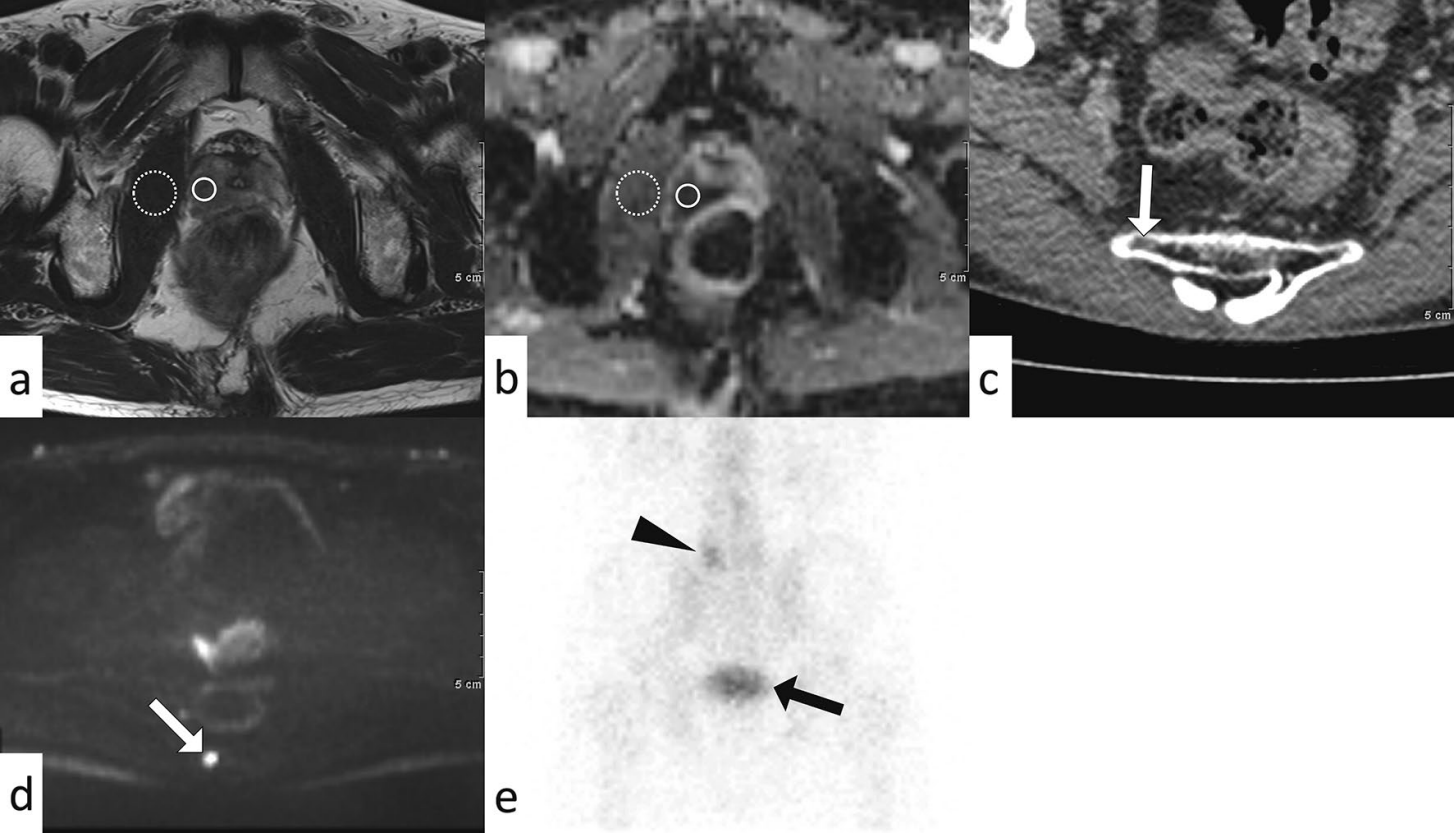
A 64-year-old man with prostate cancer with an osteolytic bone metastasis (prostate-specific antigen density = 2.5). Prostate MRI shows the primary tumor (Gleason score 9), with a normalized T2 signal intensity of 2.4 (a) and normalized apparent diffusion coefficient of 0.52 (b). CT shows an osteolytic bone metastasis in the sacrum (c, arrow), with hyperintensity on the diffusion-weighted image (d, arrow) but negativity on bone scintigraphy (e). The increased tracer uptake in the fifth lumbar vertebra is due to a degenerative change (e, arrowhead). The increased tracer uptake pointed by the arrow is the bladder (e, arrow).
